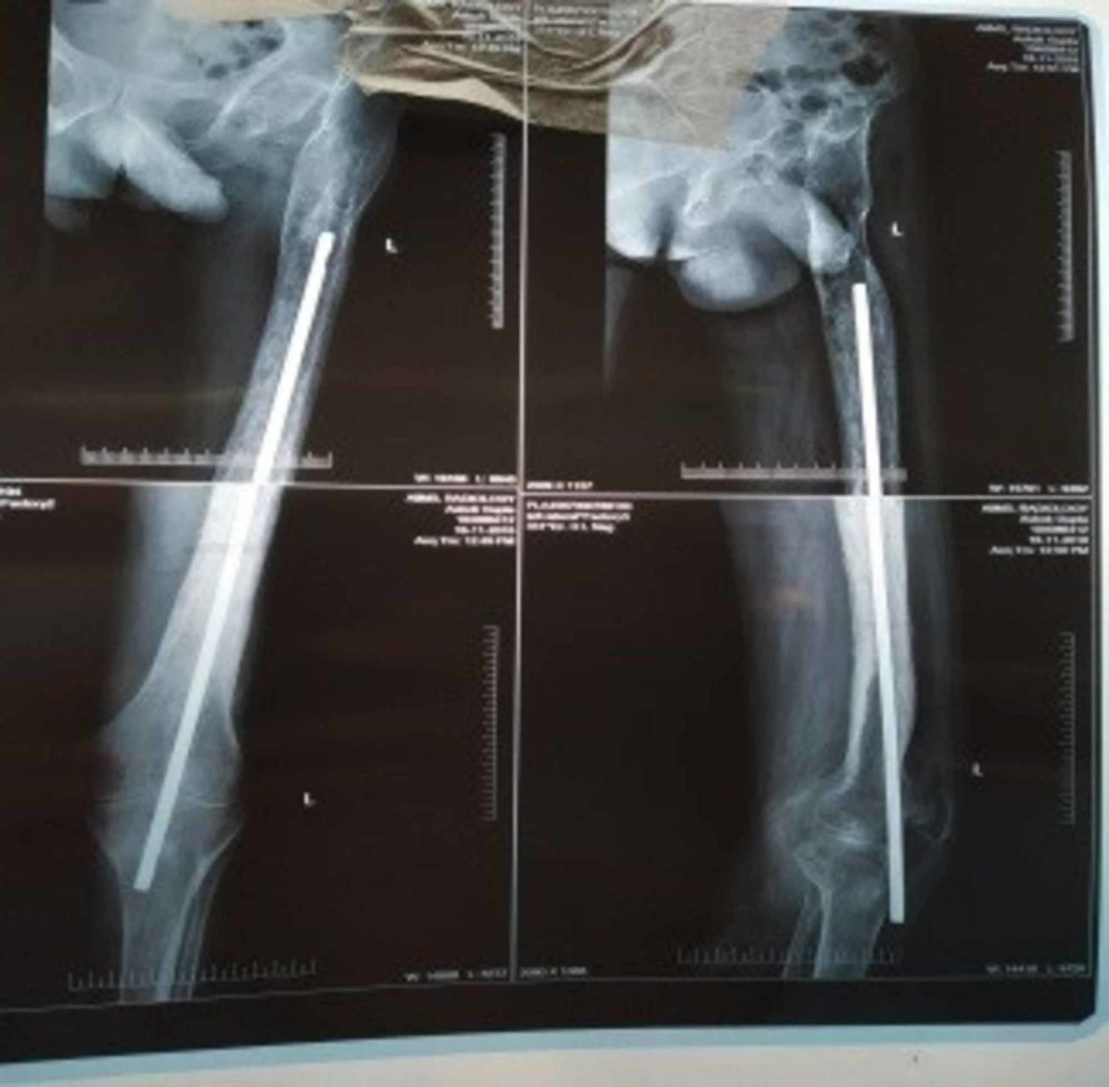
Radiograph showing distal migration of the K-nail
